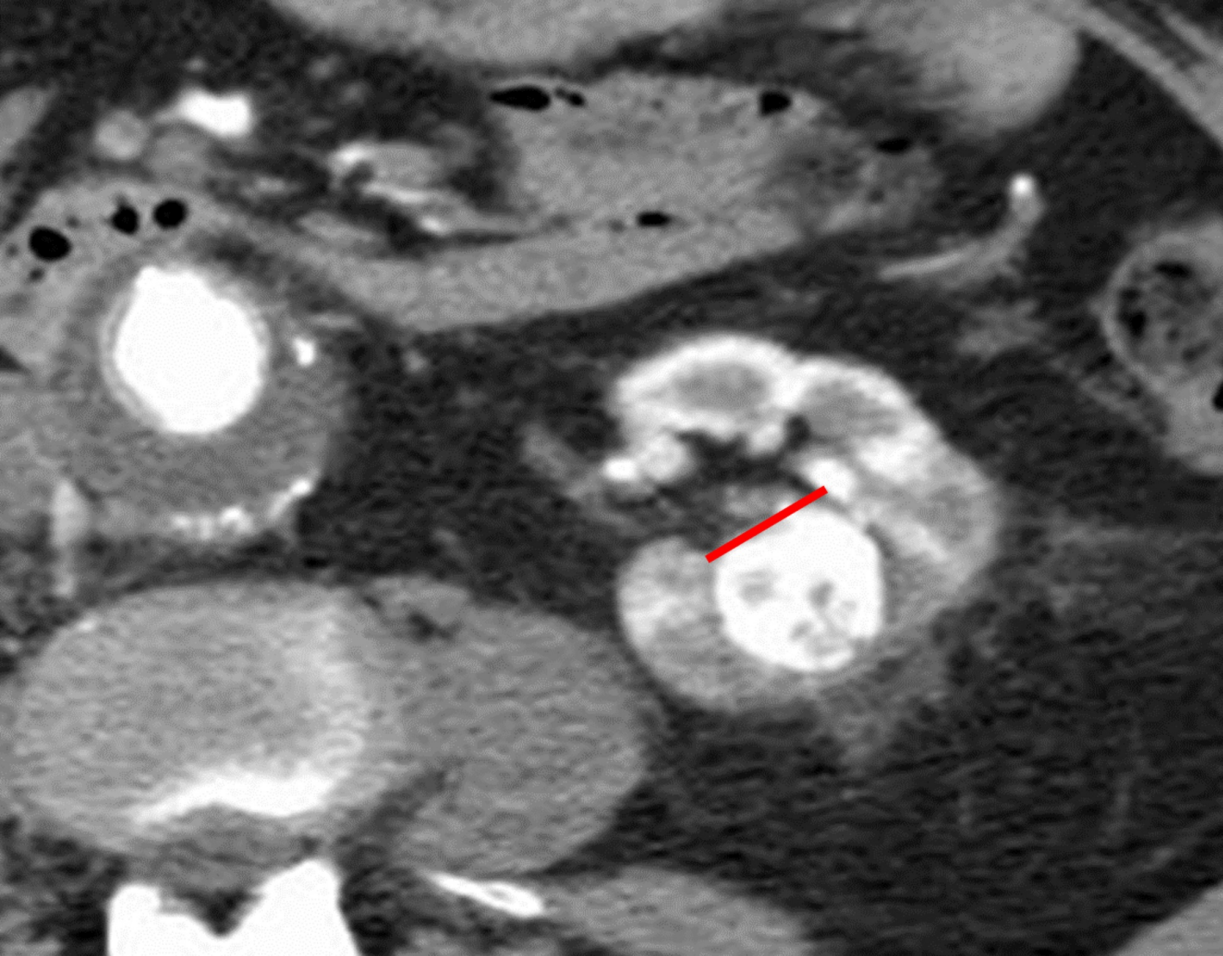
Follow-up axial CT two months post-embolization showing a 30% reduction in tumor size with loss of tumor extension into the renal pelvis (red line). Abbreviation: CT, computed tomography.
